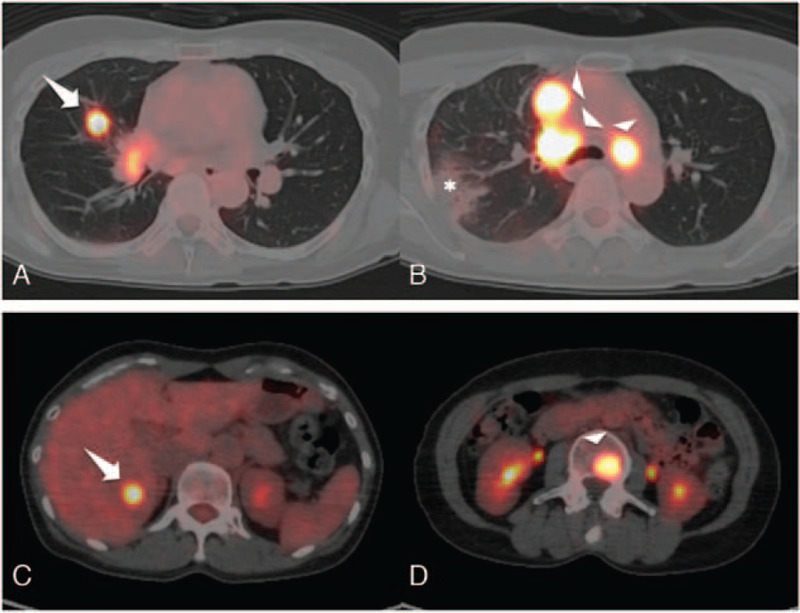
Baseline tumor burden. (A and B) Axial PET-CT image of the thorax revealing a nodule measuring 21 mm in the right middle lobe (larger diameter, arrow), consolidation in the right upper lobe (asterisk), and multiple lymph node metastases (arrowheads). (C and D) PET-CT image of the abdomen showing liver metastasis (arrow) and multiple bone metastases (arrowheads). PET-CT = positron emission tomography-computed tomography.
